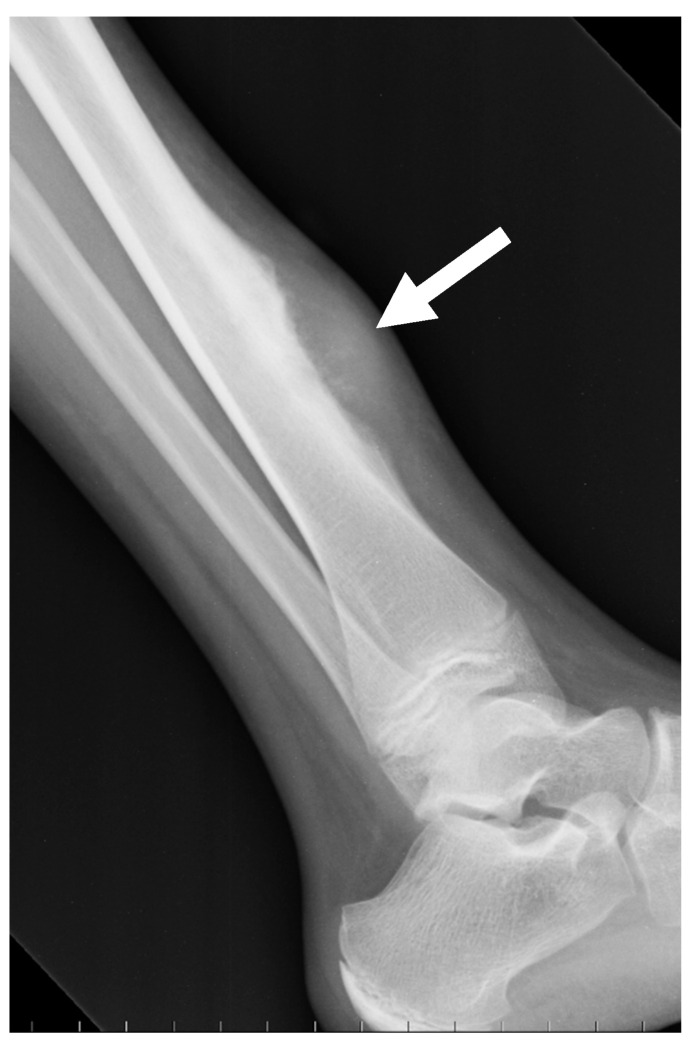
Periosteal osteosarcoma. Conventional radiography of the ankle (lateral projection) of a 13-year-old female with periosteal osteosarcoma of the distal tibia (meta-diaphyseal region); a disorganized aggressive periosteal reaction with partial superficial cortical disruption is seen on the anterior aspect of the tibia (arrow).
